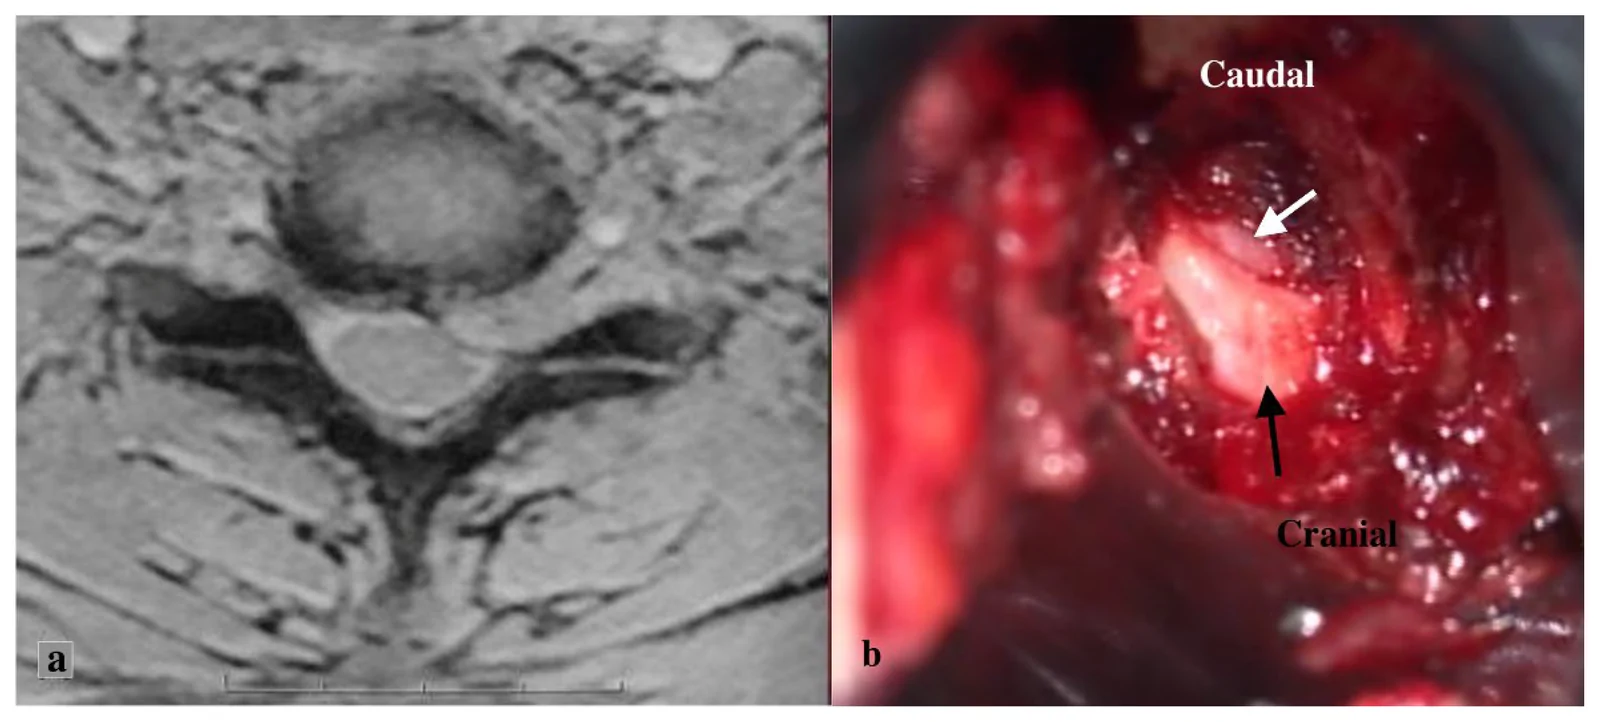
(a) Axial T2-weighted MRI image. (b) Intraoperative view showing left C6–C7 partial hemilaminectomy and foraminotomy via a posterior approach. The black arrow indicates the C7 nerve root and the white arrow indicates the conjoined nerve root located caudal to the C7 nerve root.
